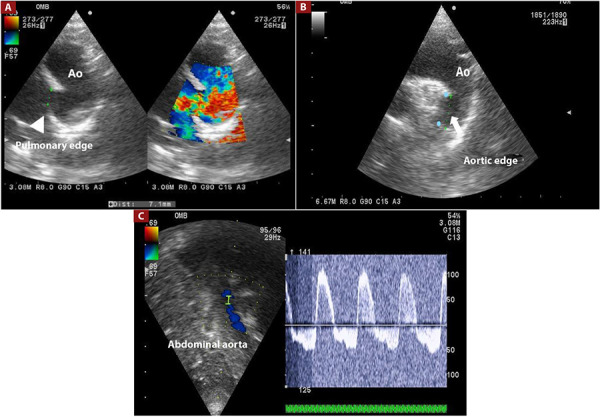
(A) Left parasternal view for the measurement of the pulmonary end of the PDA, (B) Left parasternal view for the measurement of the aortic end of the PDA, (C) Doppler spectrum at the abdominal aorta indicating the impact of the PDA. PDA: patent ductus arteriosus
